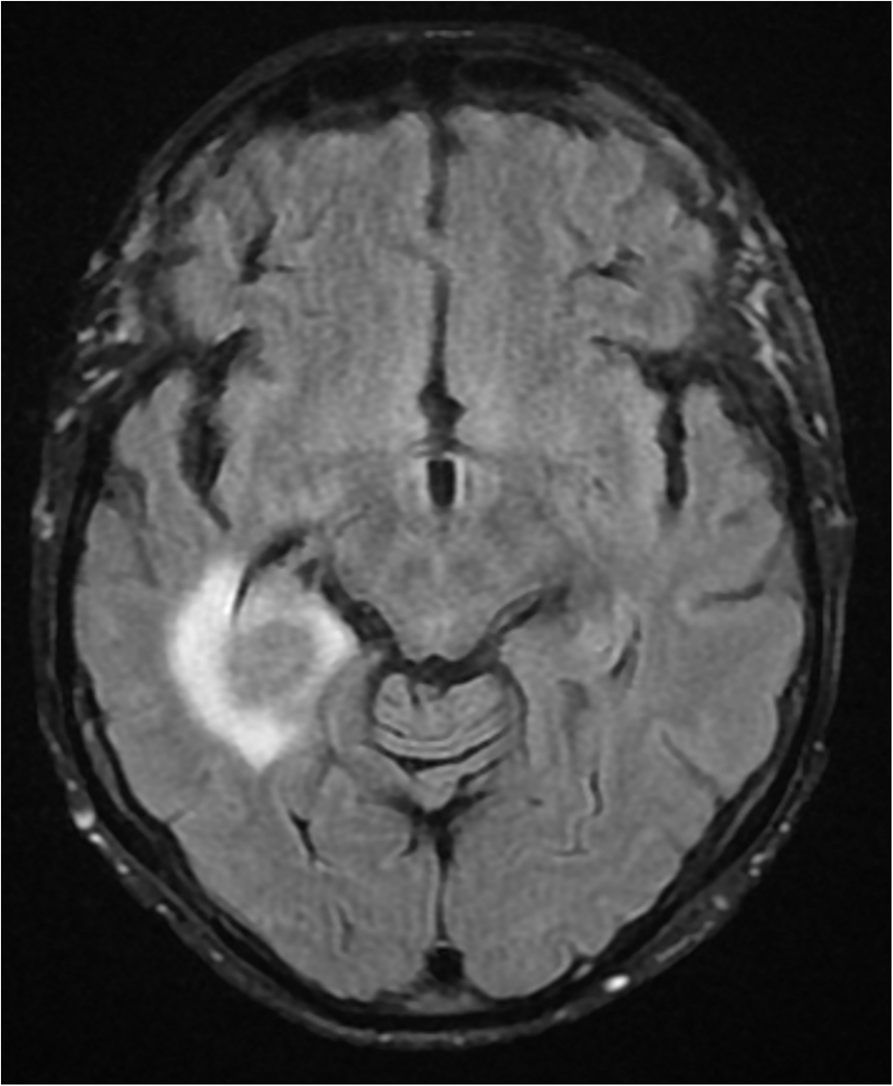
MRI of the head showing ring enhanced lesion in the right temporal lobe with surrounding edema at the time of diagnosis
